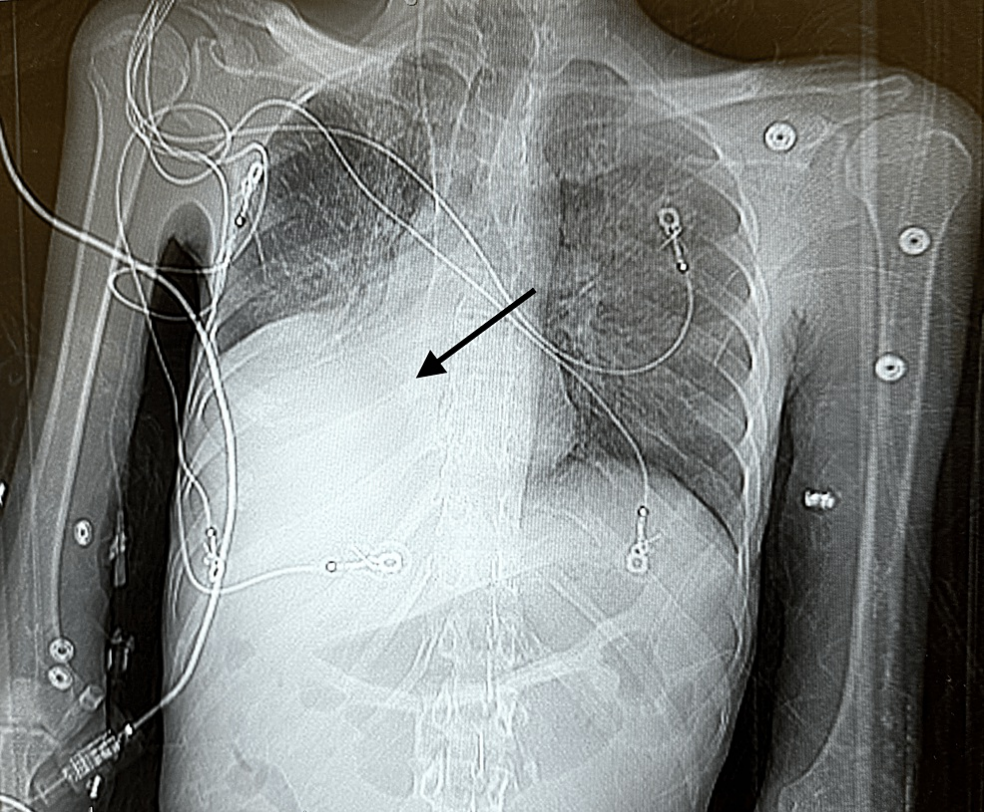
Chest CT without contrast The image displays a chest CT, and the arrow indicates a right lower lobe consolidation and a right-sided pleural effusion.
